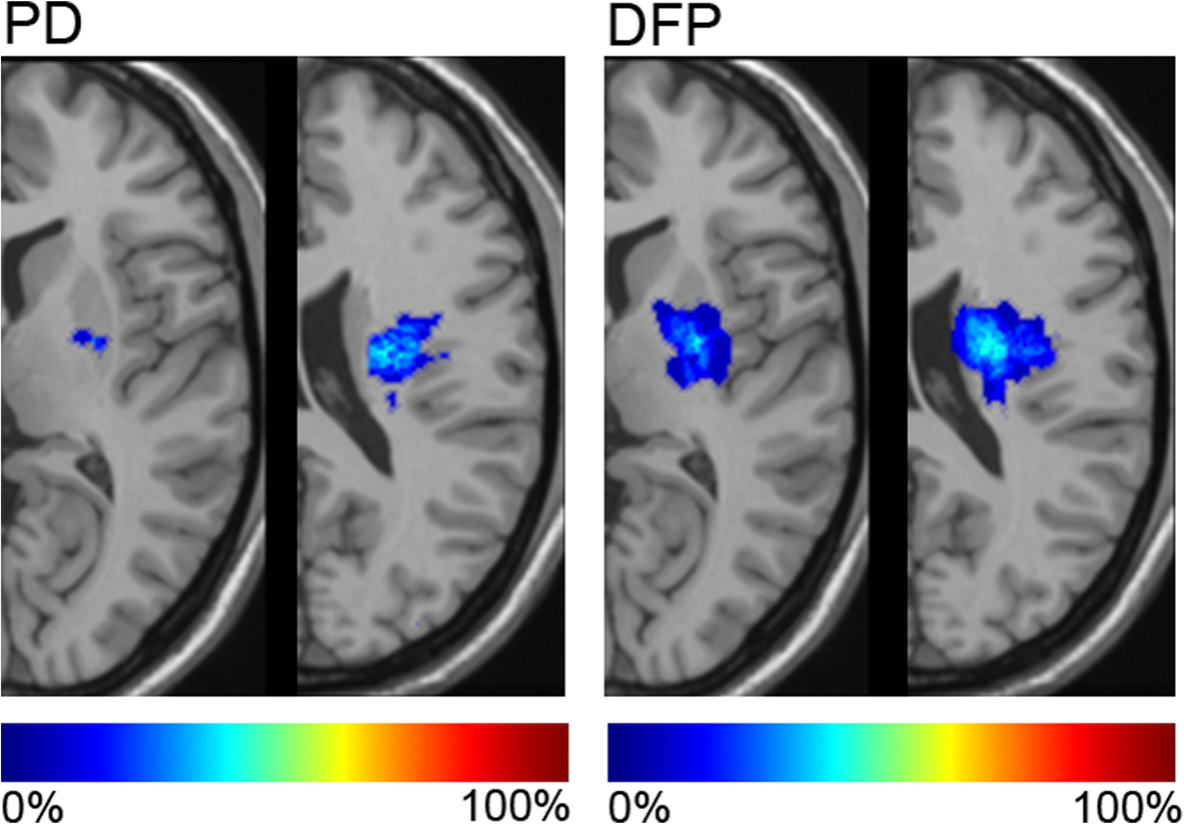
Color-coded lesion overlay plots. Lesion plots calculated from DWI images were superimposed on T1-weighted templates. Each axial slice at the level of internal capsule (left) and corona radiata (right) was given for PD (pure dysarthria) and DFP (dysarthria-facial paresis syndrome). The lesion side was not distinguished
